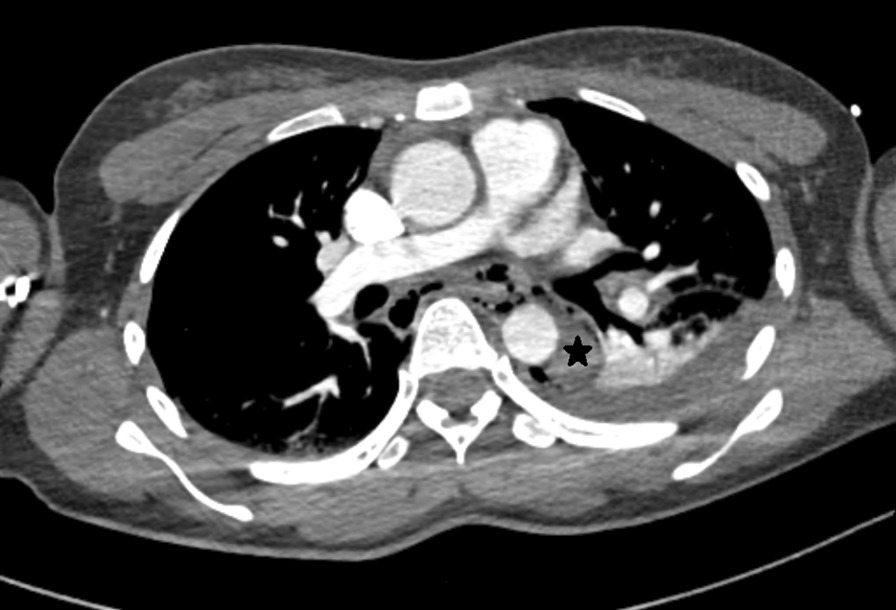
Chest CT on admission revealing abnormal fluid collection surrounding descending thoracic aorta (*)
